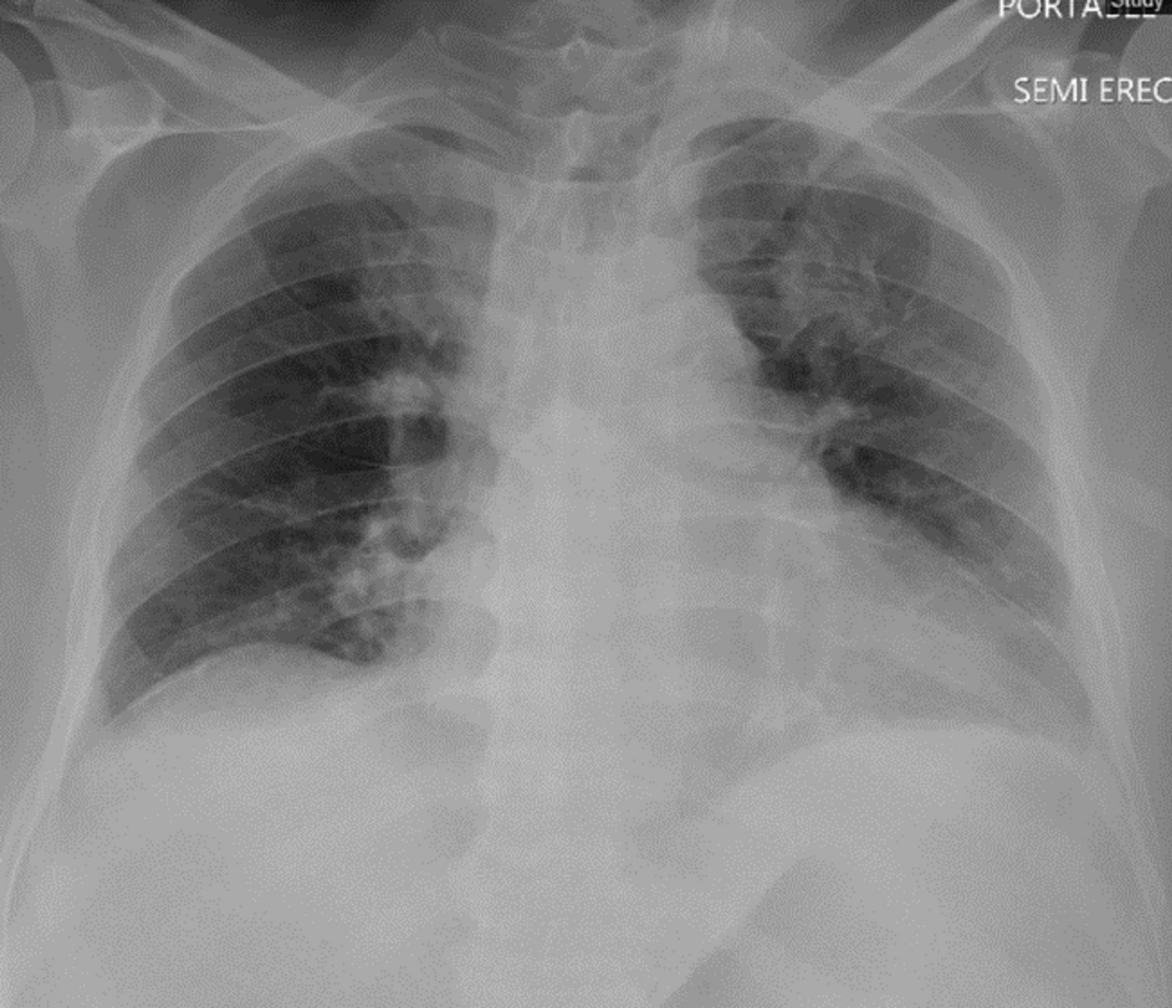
Initial chest X-ray demonstrating a subtle left upper lobe infiltrate
